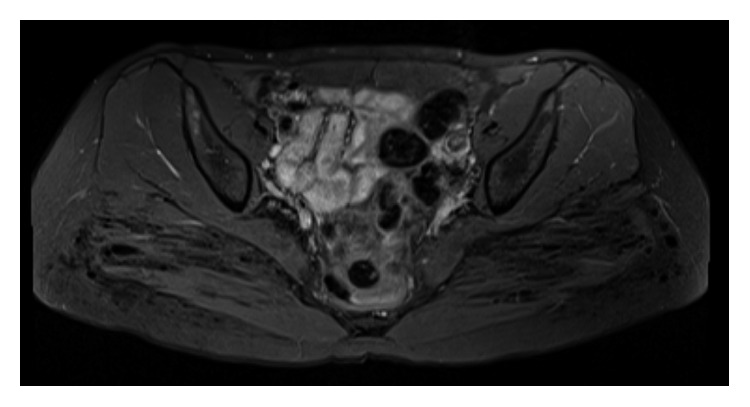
MRI of pelvis showing innumerable small low signal foci throughout the gluteus maximus and overlying subcutaneous fat bilaterally.
